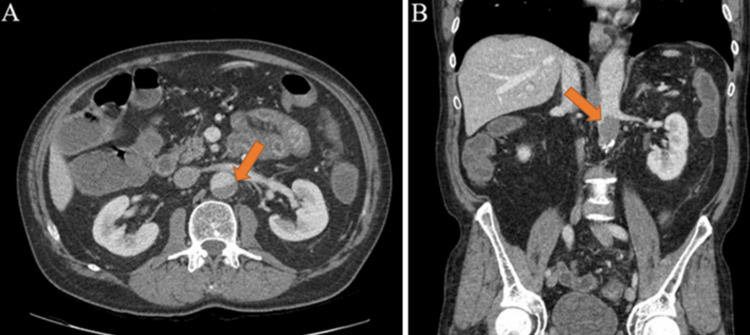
CT of the abdomen/pelvis showing aortic thrombosis (arrows) two weeks after beginning combination chemotherapy with capecitabine-oxaliplatin. A: Axial view. B: Sagittal view.
